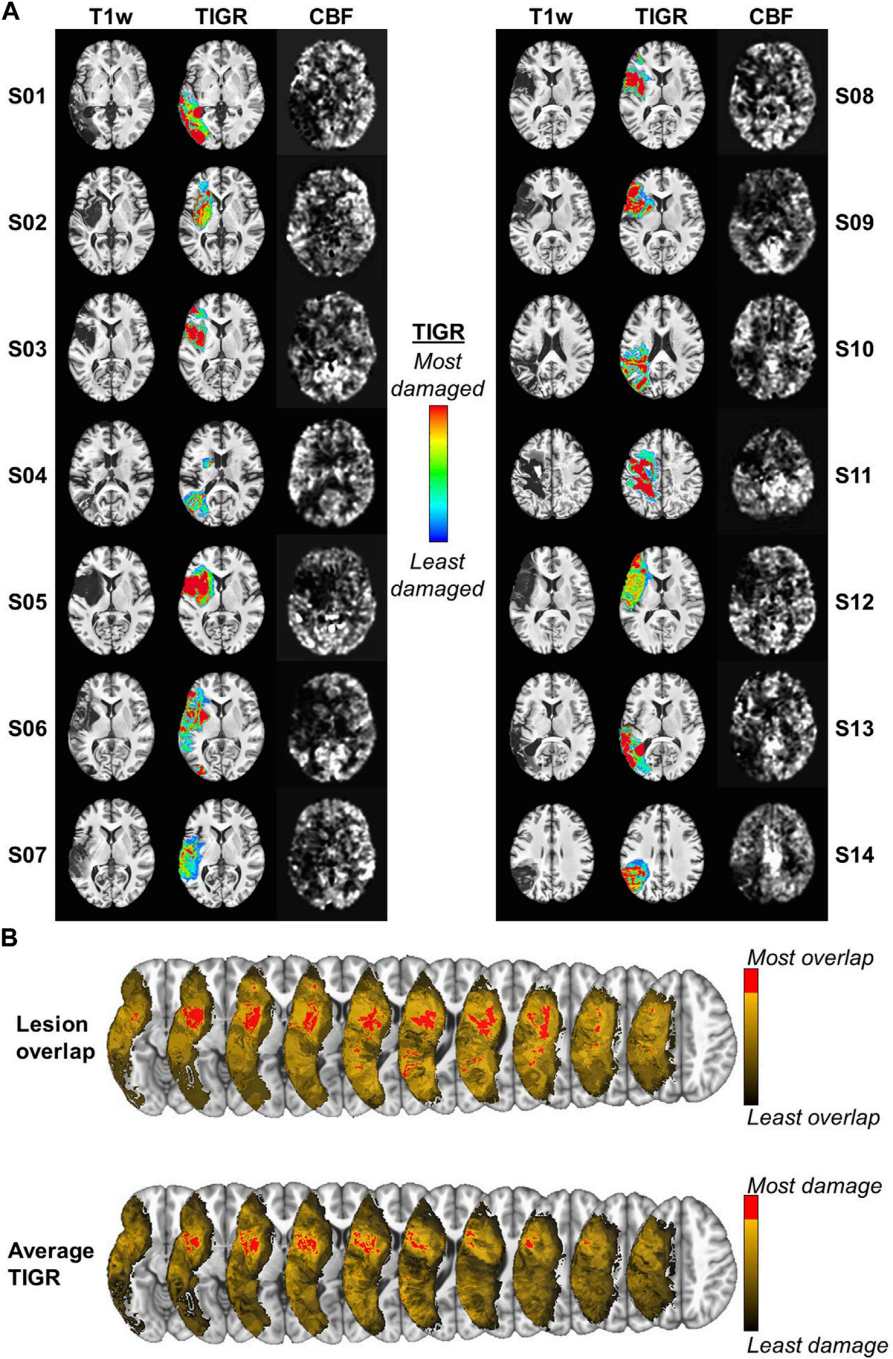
(A) The T1w, TIGR MRI, and CBF map from each individual participant, showing the heterogeneity of lesion location, tissue damage, and CBF maps. (B) The maximum lesion overlap and the maximum average TIGR scores are in adjacent, but only partially overlapping, areas. Note: Left is left in the images.
